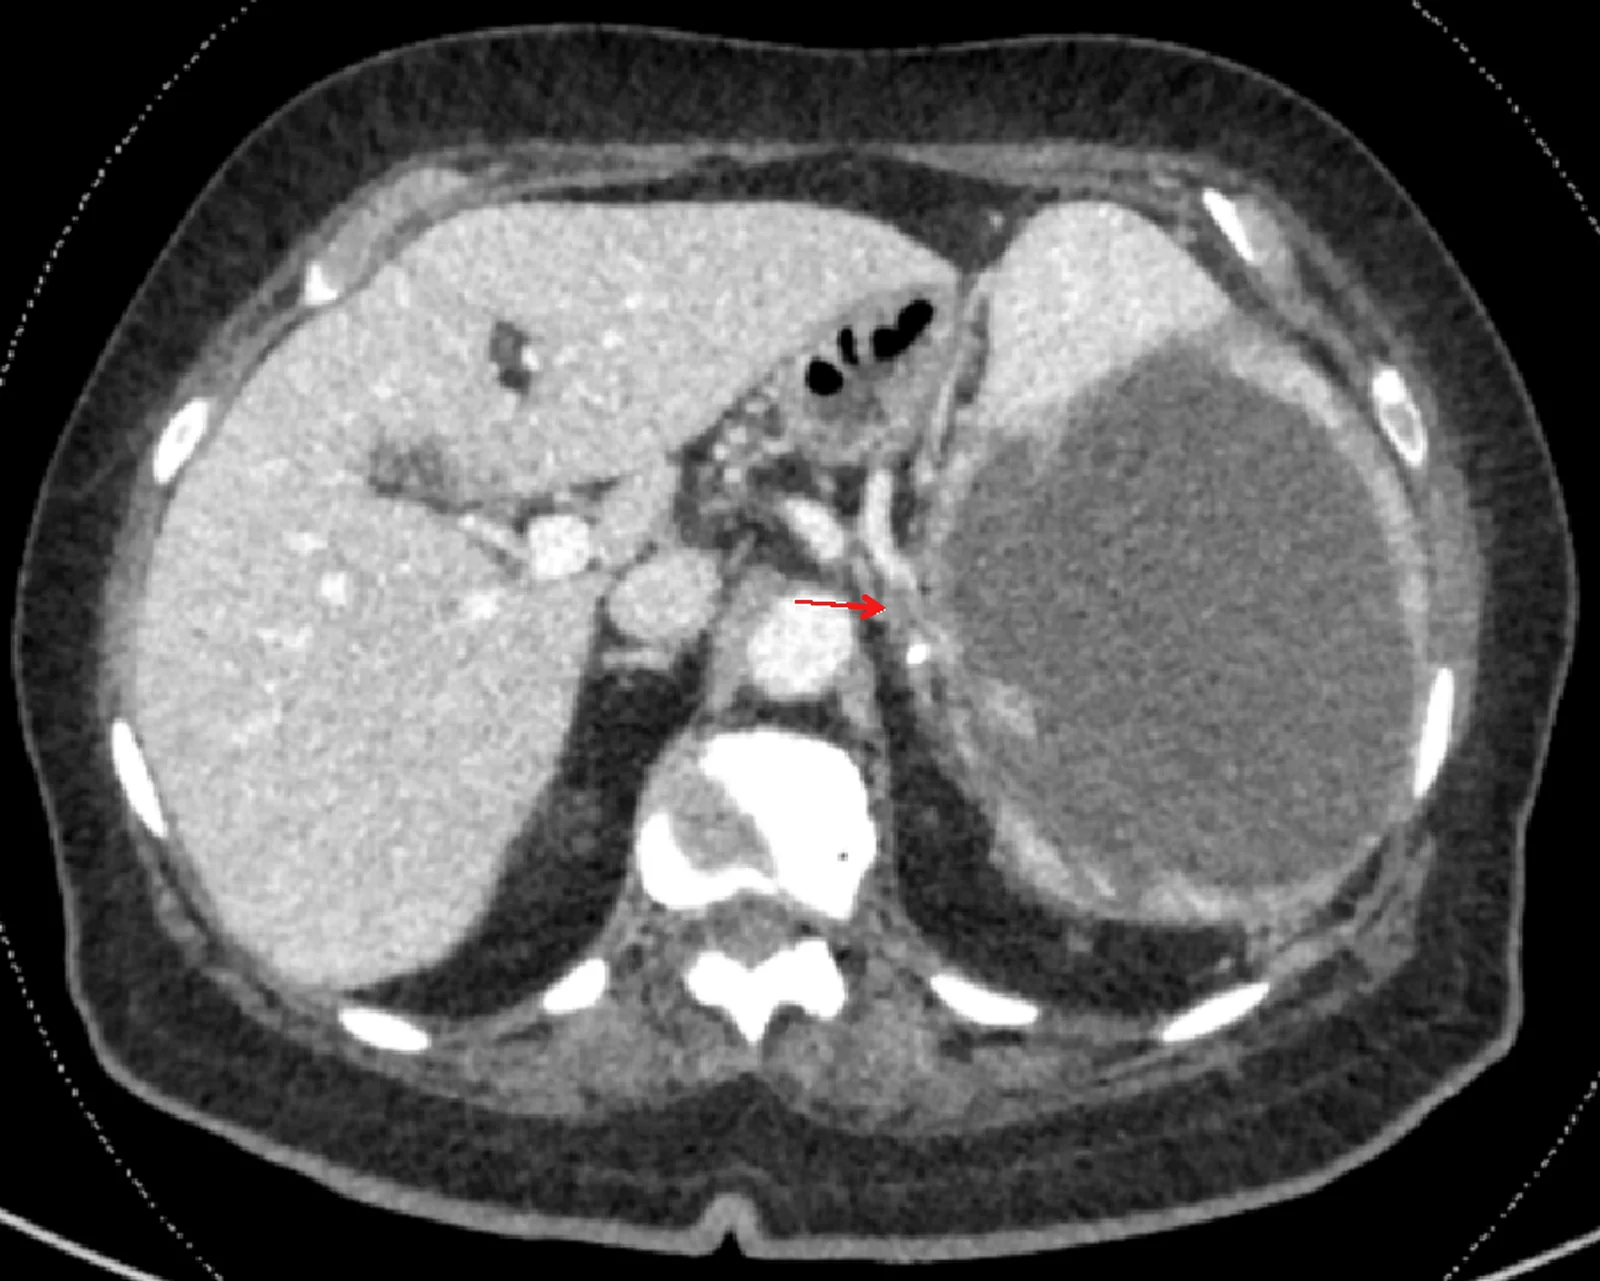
Transversal section of thoraco-abdominal CT scan in portal phase; interruption of the splenic artery indicated by an arrow
